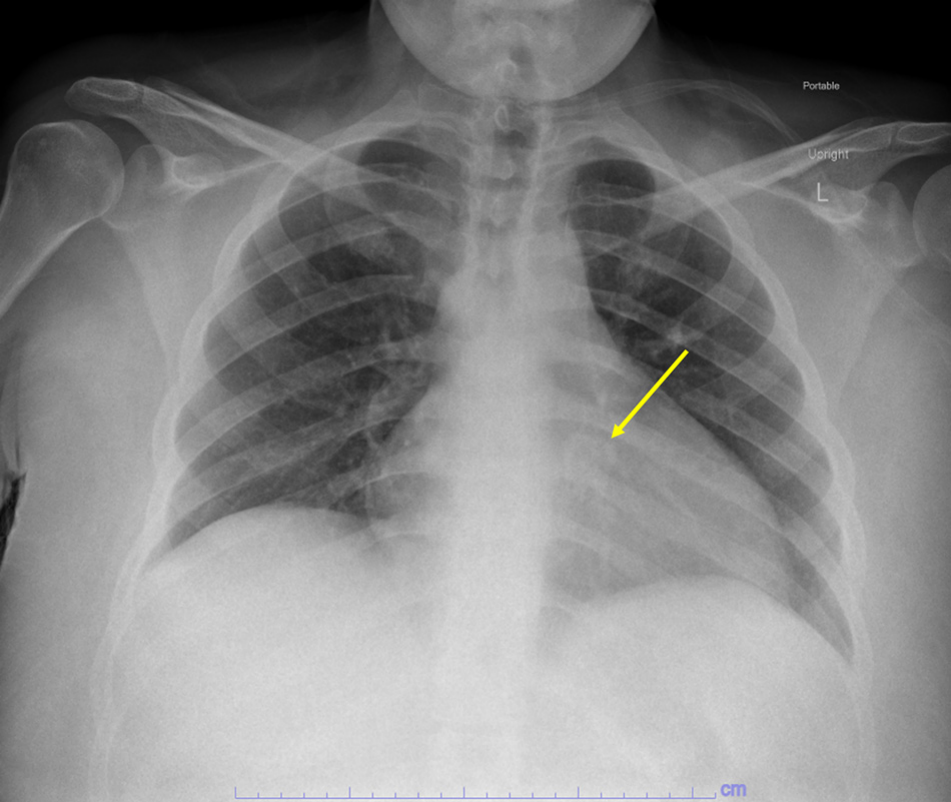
Chest Radiograph was initially reported as no acute process. However, on subsequent review, a calcified retrocardiac mass was seen (Yellow arrow) (Color version of figure is available online)
